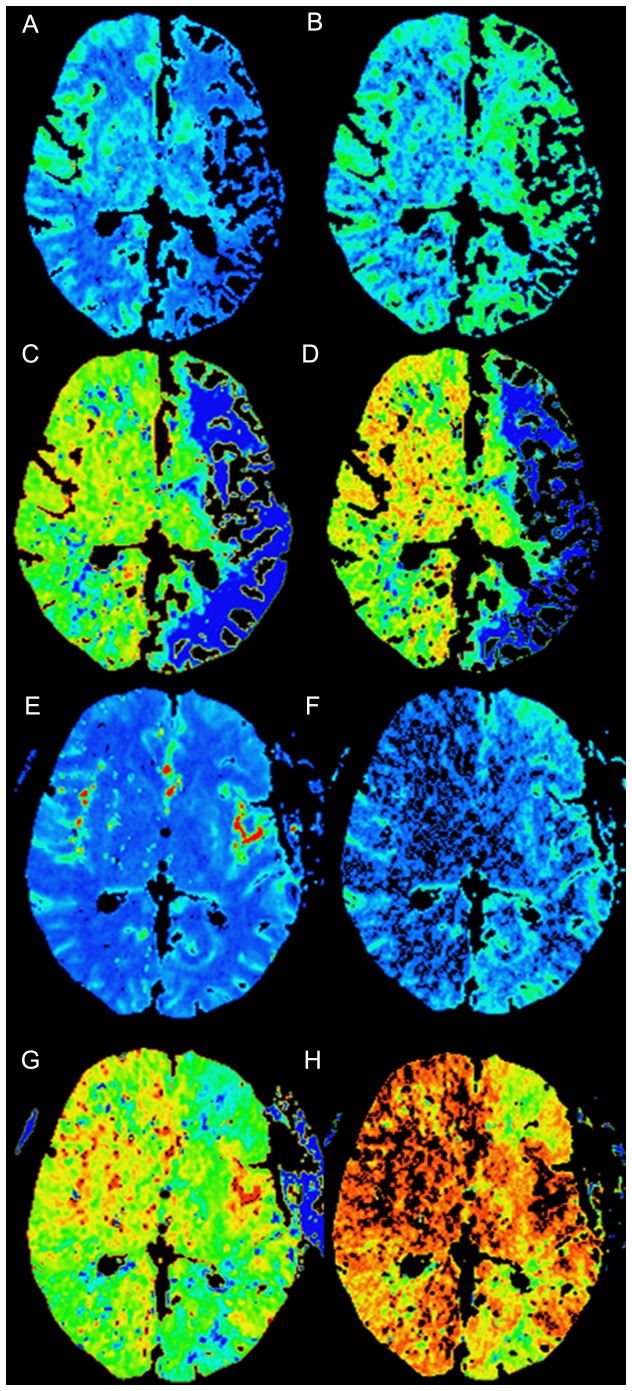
CT perfusion images before and after surgery. Images show cerebral hemodynamics changes of pre- and post-operation of a left-sided superficial temporal artery-to-middle cerebral artery anastomosis in Moyamoya disease. Axial perfusion CT images show reduced (A) cerebral blood flow (CBF), increased (B) cerebral blood volume (CBV), delayed (C) mean transit time (MTT), and (D) time to peak (TTP) in the region of left middle cerebral artery before surgery. The postoperative whole-brain perfusion CT images show increased (E) CBF, reduced (F) CBV, (G) MTT and (H) TTP in the region of left middle cerebral artery.
